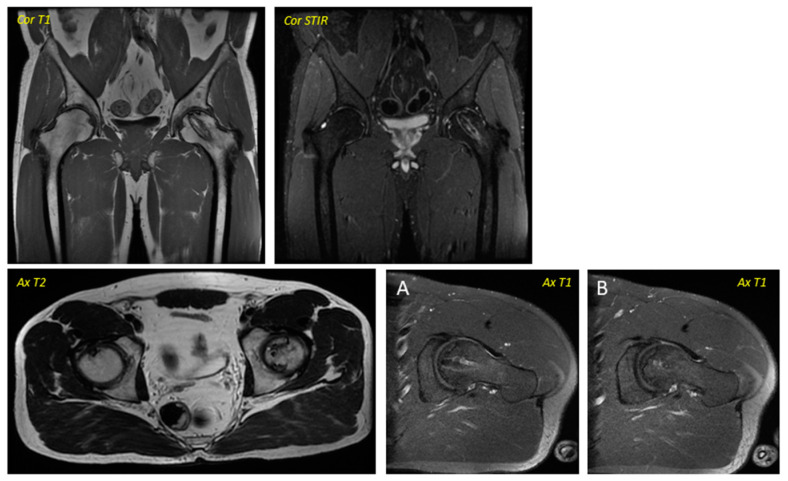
Post-surgical Magnetic Resonance Imaging corresponding to the patient in Figure 1 was performed in April 2016. Ax T1 (axial T1 plus contrast plus fat suppression–(A) Axial plus caudal incidence and axial (B) plus cranial incidence). Trocar entry is visualized in all images.
